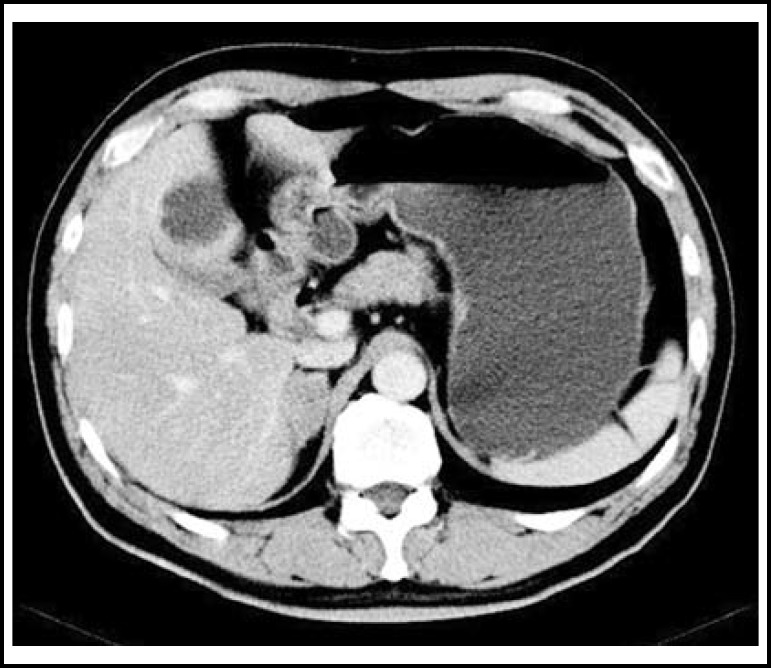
Enhanced CT scan demonstrating a right adrenal mass of low density
